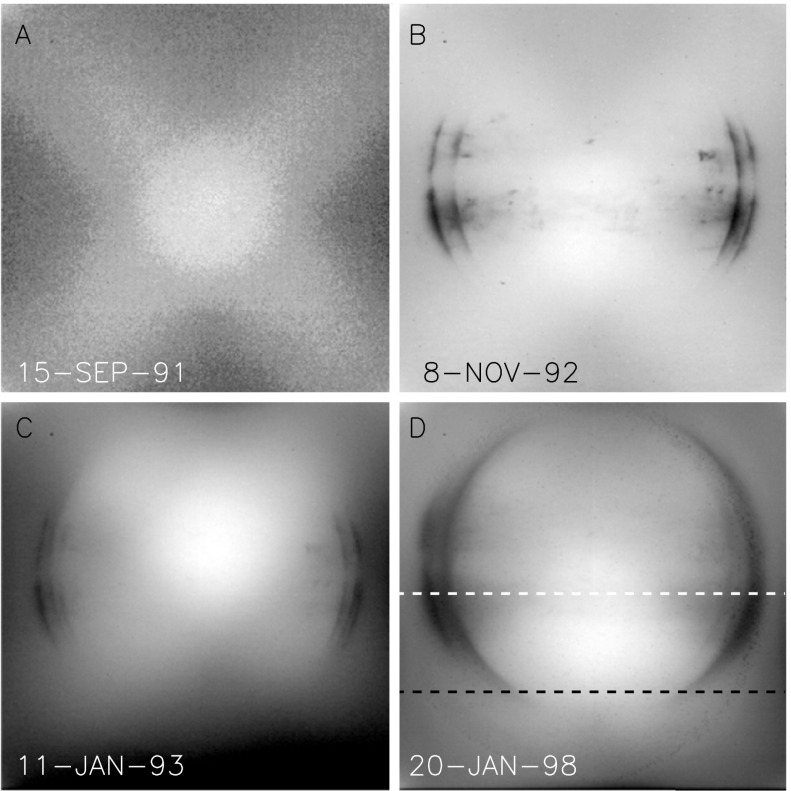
Examples of diffuser images throughout the mission, individually scaled to reveal details. (A) first diffuser image. Noisy because of eight bit compression. (B) shortly before 13 November 1992 entrance filter failure. Damage from X-ray exposure is evident. (C) two months after filter failure. About 8 % of X-ray entrance ring is open. (D) five years after (C). About two-thirds of X-ray entrance ring is open by this time. The image section between the broken black and white lines denotes the area used for producing the mission-long light curves of Figures 28 and 29.
